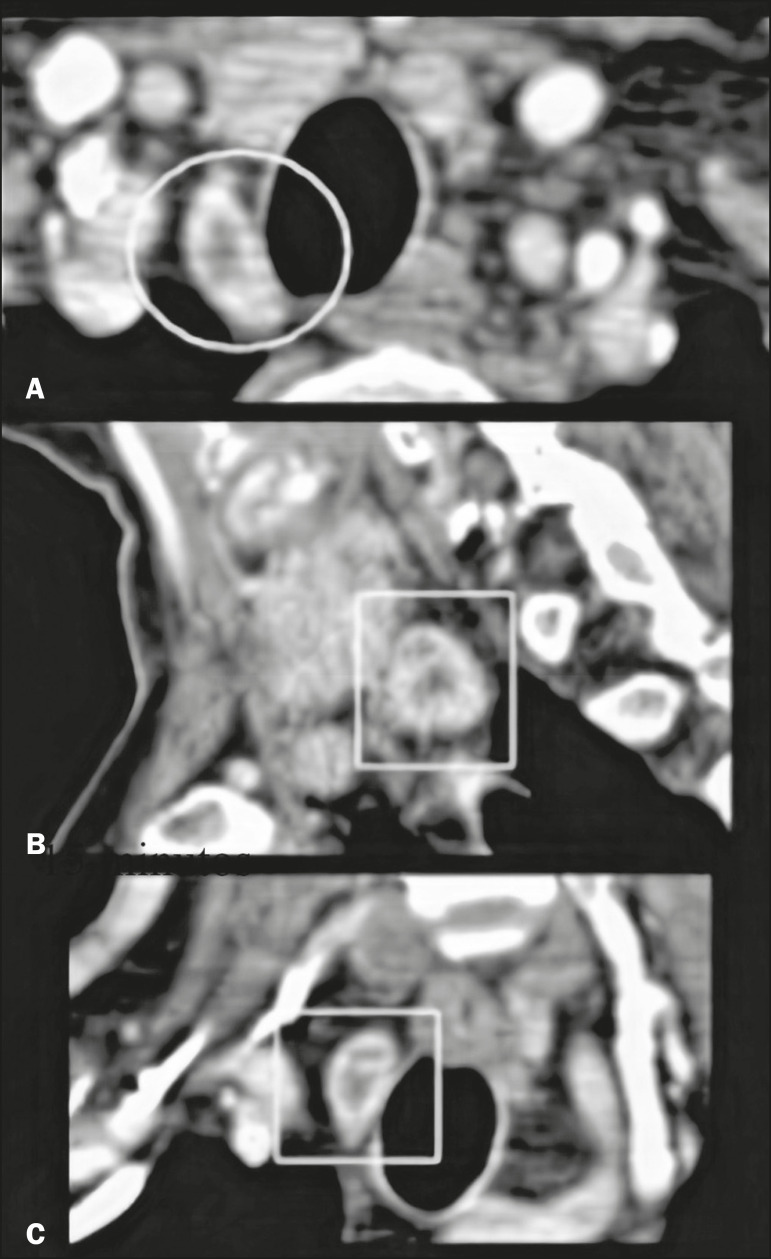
Parathyroid adenoma evaluated with the 4D CT protocol, shown in different reconstructions. A: Image of a hyperattenuating lymph node in the arterial phase, showing avid contrast enhancement (circle). In larger lesions such as this one, enhancement is not always homogeneous. B: Image showing the nodule (rectangle) in the sagittal plane. C: Image showing the nodule (rectangle) in the coronal plane.
